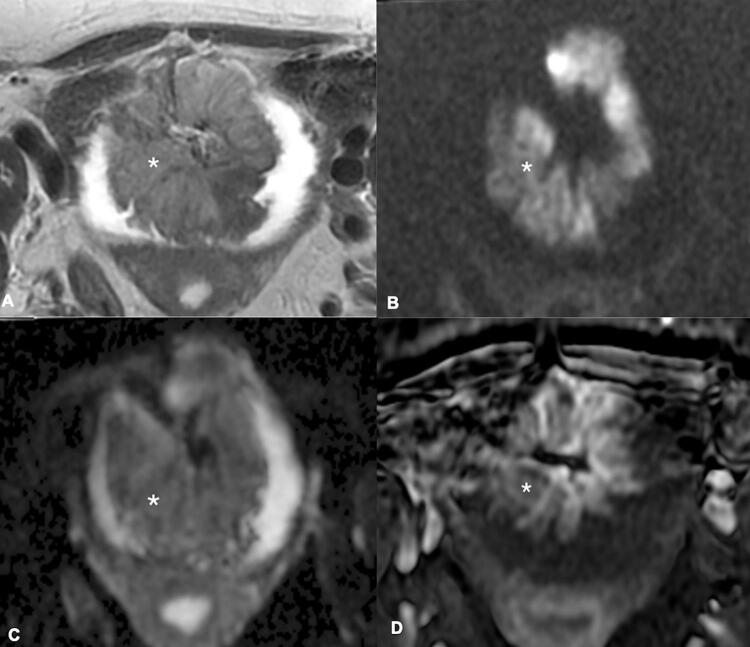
A 72-year-old woman presented with macroscopic hematuria. A) T2W image (axial plane) shows a large stalked mass at the anterior bladder wall. B and C) DWI (b=2000) and ADC maps show significantly restricted diffusion, not extending through the muscularis propria; the “inchworm” sign can be appreciated. D) DCE imaging shows early and heterogeneous enhancement of the lesions, not extending through the muscularis propria. The final VI-RADS score was 2. T2W, T2 weighted; DCE, dynamic contrast-enhanced; DWI, diffusion-weighted image; ADC, apparent diffusion coefficient.
